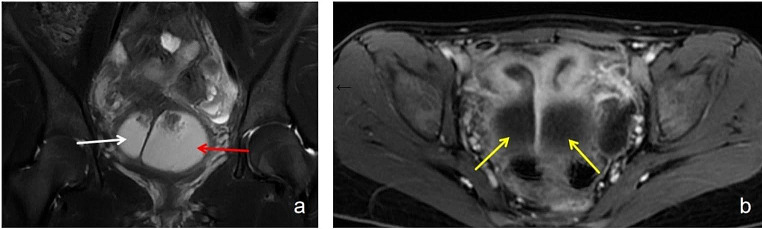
MRI of the pelvis. (a). Bilateral hematocolpos (white straight arrow suggesting hematocolpos caused by the oblique septum and red straight arrow indicating hematocolpos caused by imperforate hymen. (b). Bicornuate bicollis uterus (straight arrow suggesting bilateral hematometra). The pelvic MRI showed bicornuate bicollis uterus, with bilateral hematometra, and bilateral hemivagina (commuicating with the hemiuteru), distended by a content whose signal was methemoglobin
